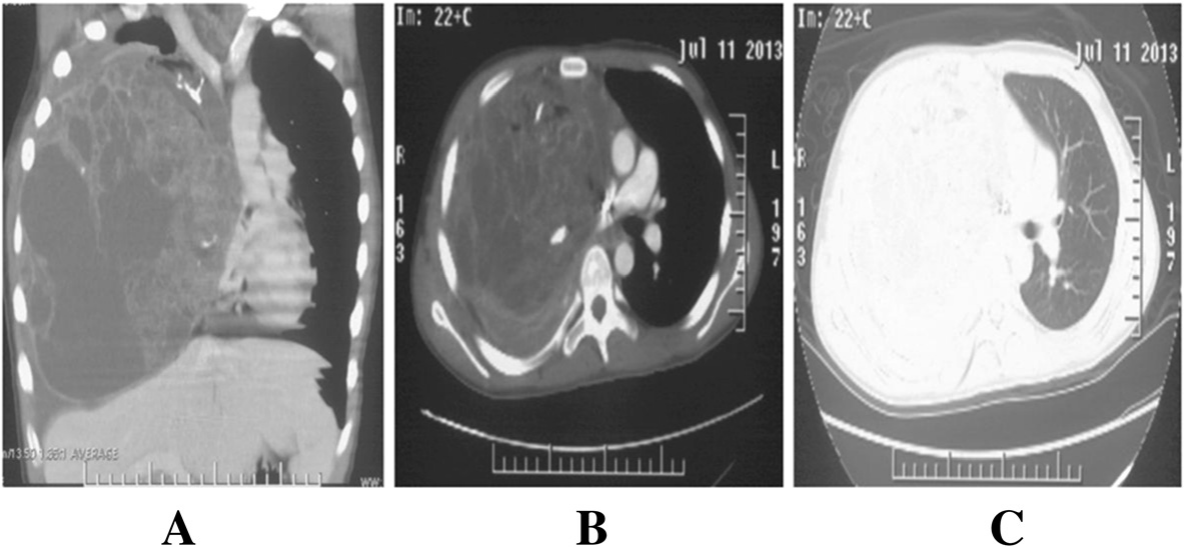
Enhanced chest CT scan before operation. (A) In the coronal mediastinal window, preoperational CT scan showing the mass compressing the mediastinal vessels, heart and airway. (B) In the mediastinal window. (C) In the lung window.
